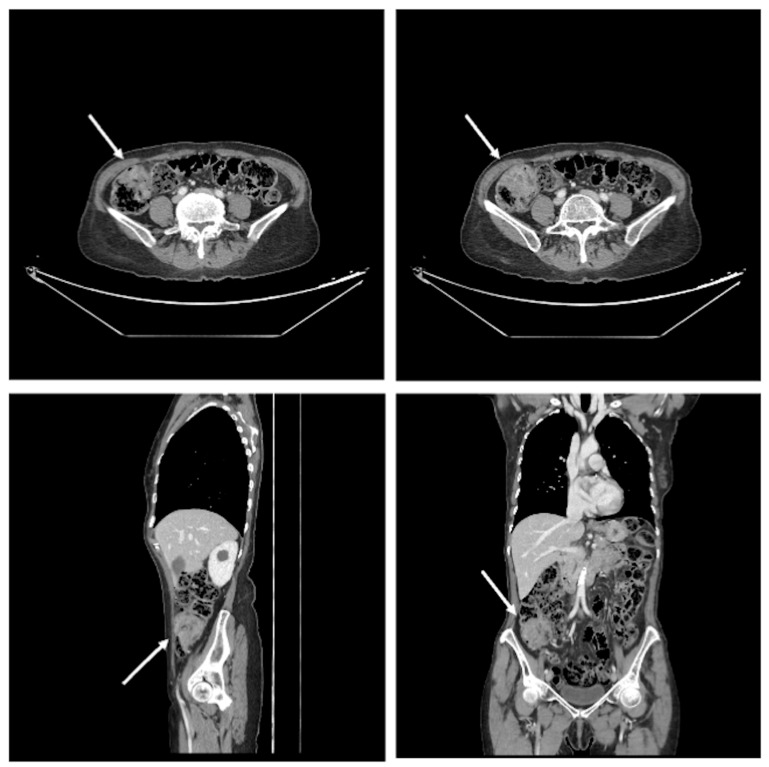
Contrast-enhanced computed tomography scan showing heterogeneous circumferential thickening of the cecum (approximately 5 cm in length), suspicious for malignancy, with small adjacent pericolic lymph nodes and no evidence of distant metastatic disease (arrows pointing to the cecal tumor).
